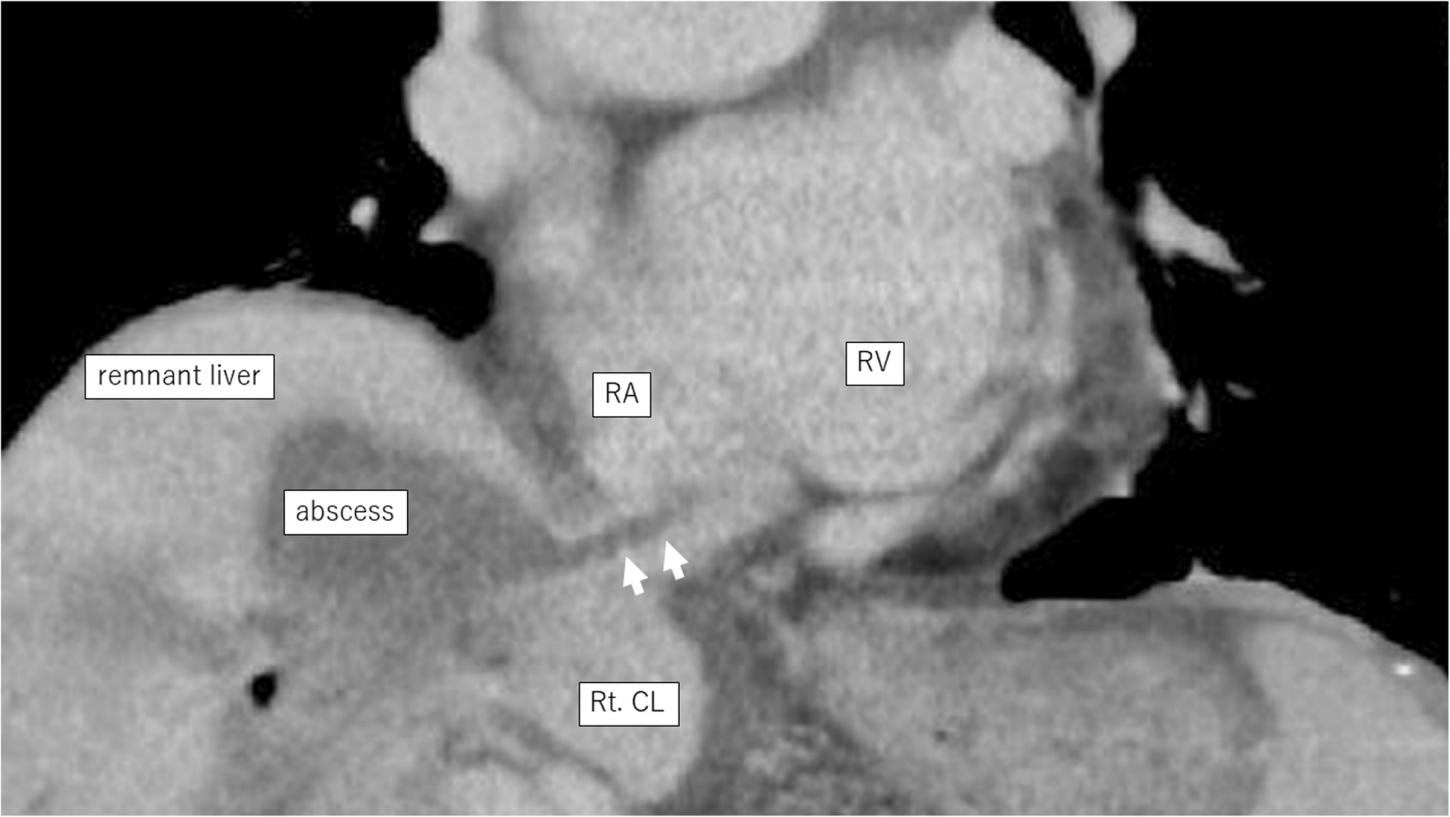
An enhanced abdominal CT scan showed the low density area continuous from the abscess to the RA (arrow). *RA* right atrium
